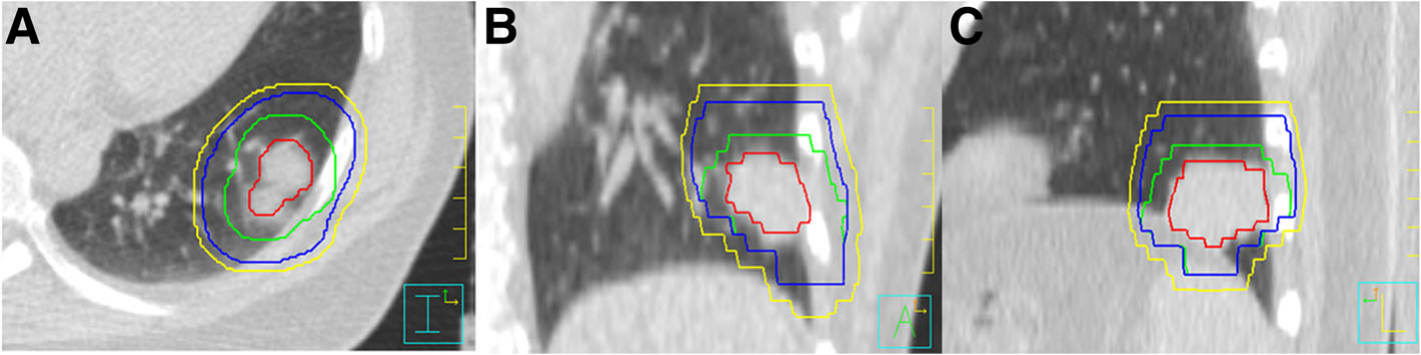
Phase 0% of four-dimensional computed tomography (4DCT) image for an protocol NSCLC patient. (a) transverse (b) sagittal (c) coronal; Gross tumor volume (GTV) of phase 0% in *Red*; Clinical target volume (CTV) of phase 0% in *Green*; Internal clinical target volume (ITV) in *blue*; Planning target volume (PTV) in *Yellow*
